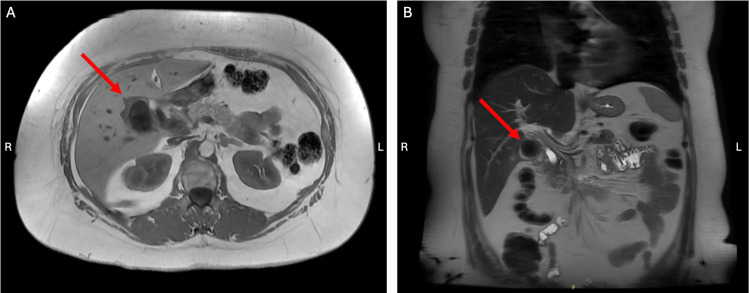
Magnetic resonance cholangiopancreatography (MRCP) Abdominal MRI demonstrating pericholecystic fluid collection and gallbladder wall thickening (red arrows) in the axial (A) and coronal planes (B).
